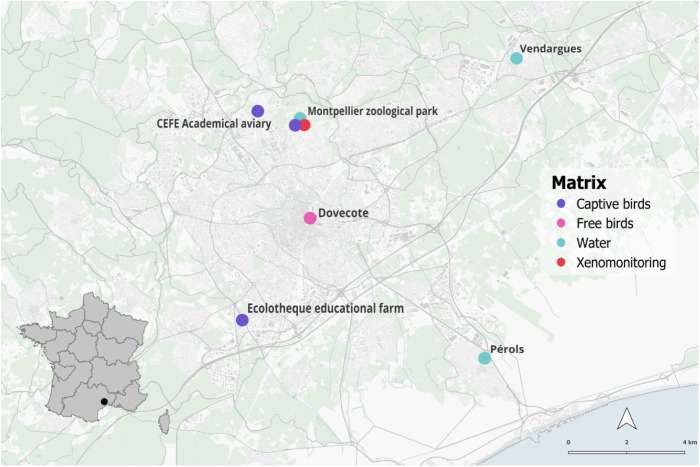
Sites, tested matrices and USUV detection in the Montpellier metropolitan area (black dot on the general map). Base map data from OpenStreetMap, via Geofabrik (https://download.geofabrik.de/), under the Open Database License (ODbL). Sea layer created by the authors. Administrative boundaries obtained from freely available open data sources.
